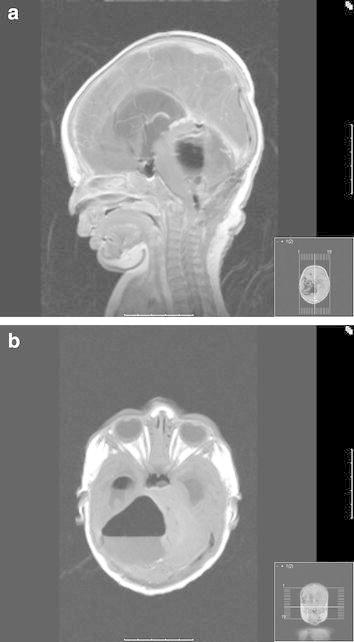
Immediate postoperative MRI scans; a sagittal, b axial, *white line* demonstrating gross total resection.
